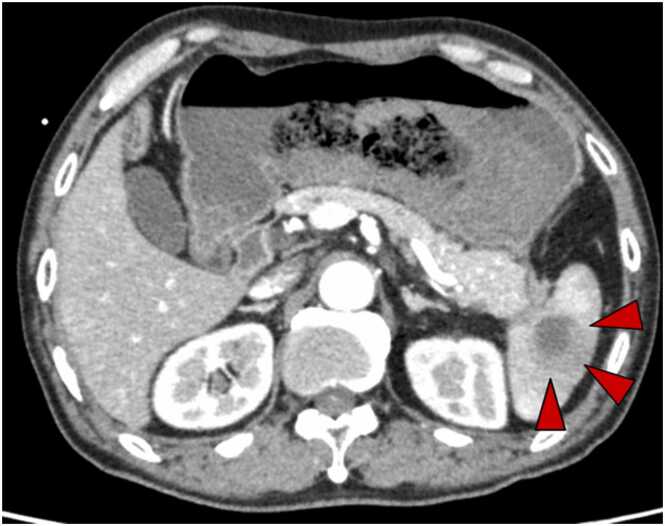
Contrast-enhanced abdominal CT at emergency department presentation revealed a 17 × 20 mm low density area within the spleen.
